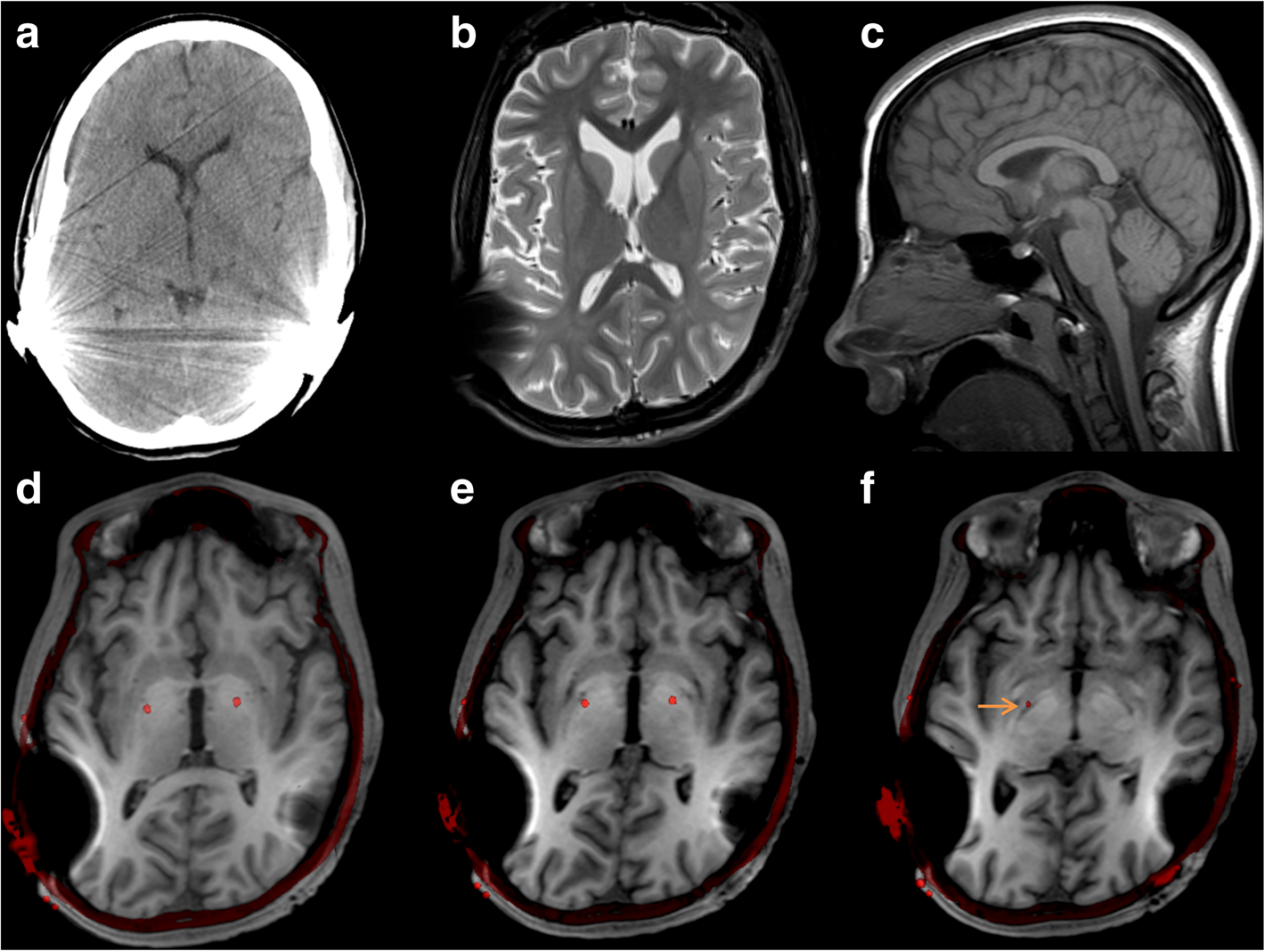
Brain CT (2009) and preoperative brain MRI (2013), fused with postoperative CT. Preoperative MRI (b–f) did not show evidence of cortical developmental malformations, corpus callosum defects, or any other inborn brain structure anomalies. The maturation of the brain including myelination is normal. Comparison of an axial CT from 2009 (a) and MRI T2 from 2013 (b) shows enlargement of the lateral ventricles, indicating some supratentorial volume loss over time. A mid-sagittal T1 (2D TSE) image from 2011 (c) shows normal configuration and no certain volume loss of the brain stem or cerebellum, and the preoperative MRI series (2013) did not disclose convincing signs of infratentorial volume loss (not shown due to more distorted images). Images d–f show preoperative T1-3D MPRAGE* (2013) fused with postoperative CT images (2015), showing the position of the DBS electrodes visualized as red dots (image fusion performed using software from NordicICE, NordicNeuroLab, Bergen, Norway). Images d and e show the electrodes in the posterior third and most ventral part of the internal globus pallidum. Image f shows the right electrode tip touching upon the optic tract (orange arrow) (*3D T1 MPRAGE was used for fusion with postoperative CT instead of T2 (which better visualizes the basal ganglia), because the cochlea implants inflicted heavy susceptibility artifacts on the T2 images with pronounced focal signal loss, as well as distortion. Also, the CT alignment/co-registration with the large (whole head) field of view 3D T1 MPRAGE series was accurate, whereas the CT alignment with the (reduced field of view) T2 images was not.)
